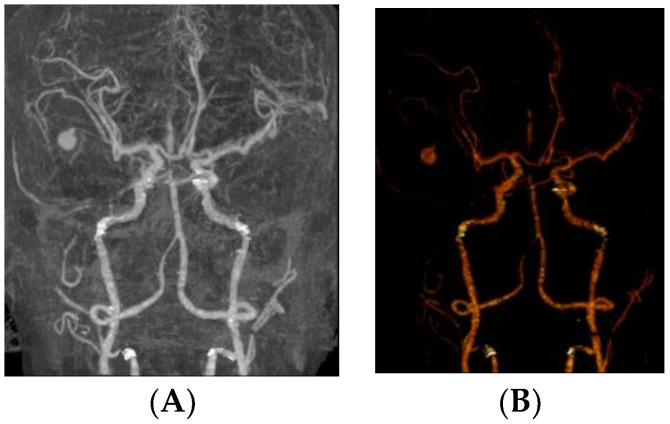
Computed tomography angiography before (A) and after reconstruction (B), showing a suspicious pseudoaneurysm without connection to an intracranial vessel.
